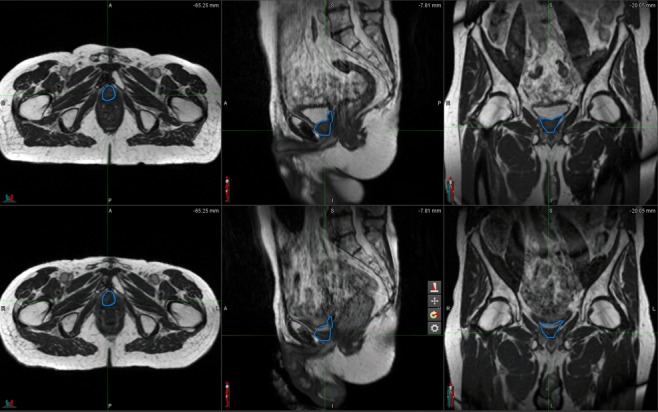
Initial and 30-month Follow-up ViewRay Magnetic Resonance Imaging of the Recurrent Disease The top row presents the initial ViewRay (ViewRay, Inc., Cleveland, OH, USA) system magnetic resonance imaging (MRI) in three planes, with the gross recurrence outlined in blue. The second row presents a 30-month follow-up ViewRay system MRI in three plan, with the same blue contours from the initial plan overlaid. This demonstrates a significant radiological response.
